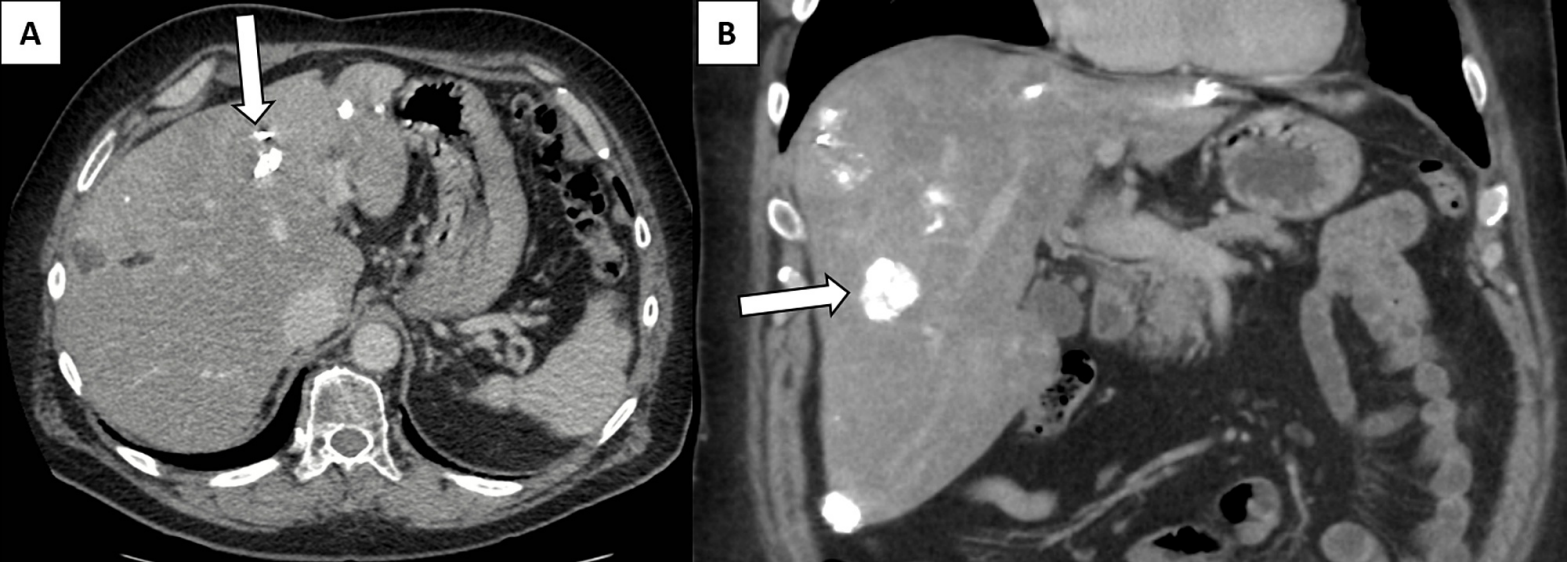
The most recent CT of patient after four years from the initial diagnosis and 11 transarterial chemoembolization procedures. The axial (A) and Coronal (B) planes demonstrate the treated multifocal hepatocellular cancer taking up Lipiodol with resolution of left sided disease.
